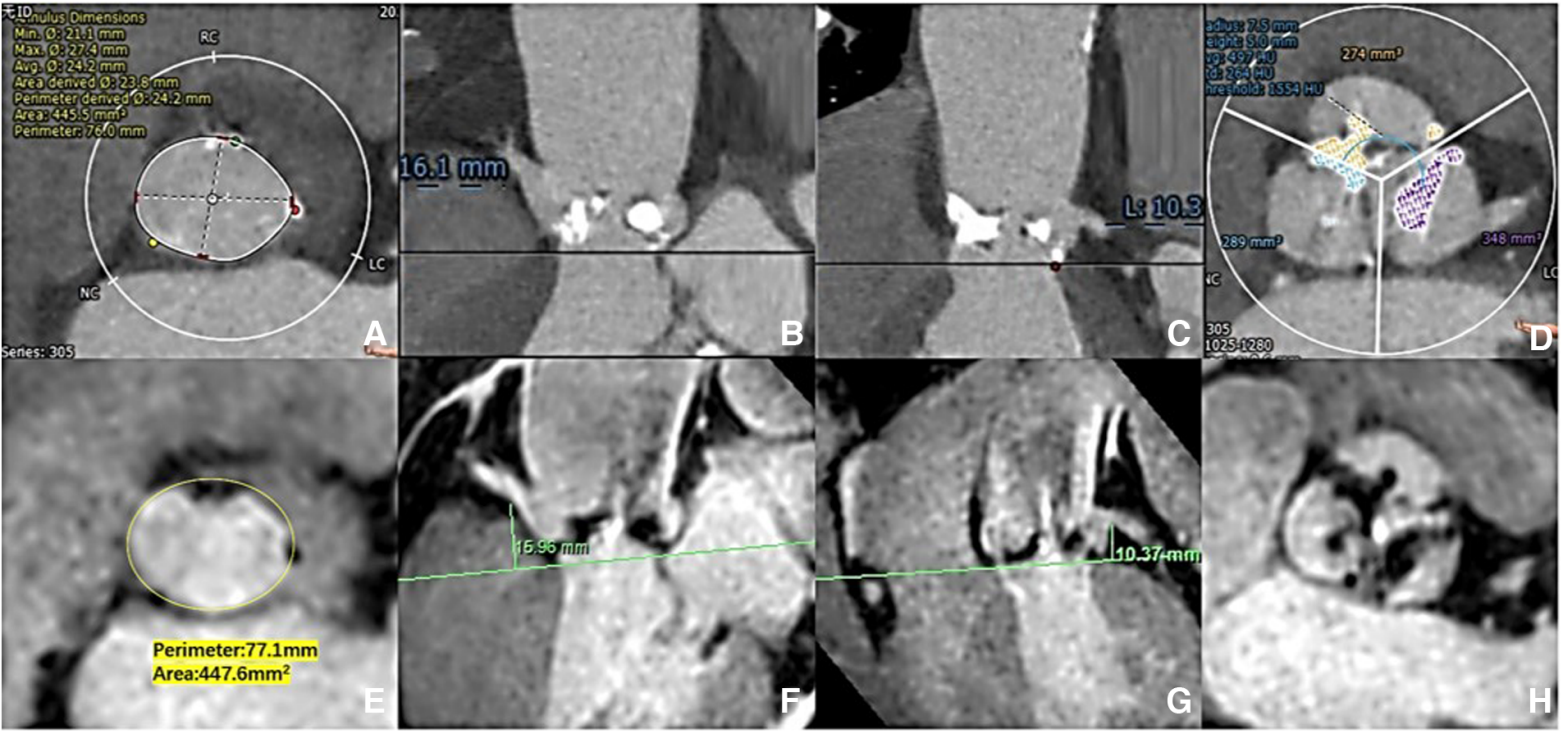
Aortic root measurement: CCTA vs. 3D whole-heart MRI. The aortic ring dimensions were assessed [(A) CCTA vs. (E) 3D whole-heart MRI]. The right/left coronary ostial heights were measured perpendicular to the annulus plane [(B,C) CCTA: vs. (F,G) 3D whole-heart MRI]. The extent of valve calcification was assessed [(D) CCTA vs. (H) 3D whole-heart MRI).
